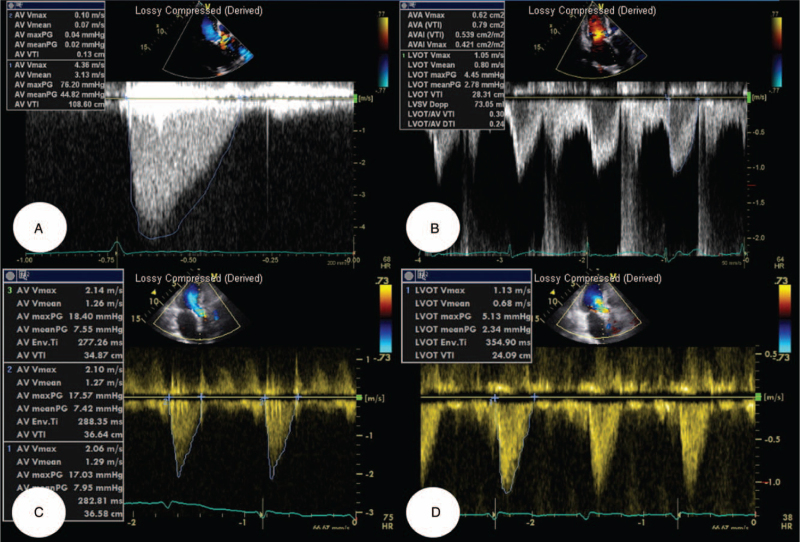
(A-B) Before TAVR, transthoracic echocardiogram was performed, demonstrating a peak velocity was 4.36 m/s, and a mean pressure was 44.8 mmHg in AoV, suggestive of severe AS. (C-D) After successful transsubclavian TAVR, a peak velocity was dropped to 2.06–2.14 m/s, with a reduction of mean pressure to 7.42–7.95 mm Hg. AoV = aortic valve; AS = aortic stenosis; TAVR = transcatheter aortic valve replacement.
